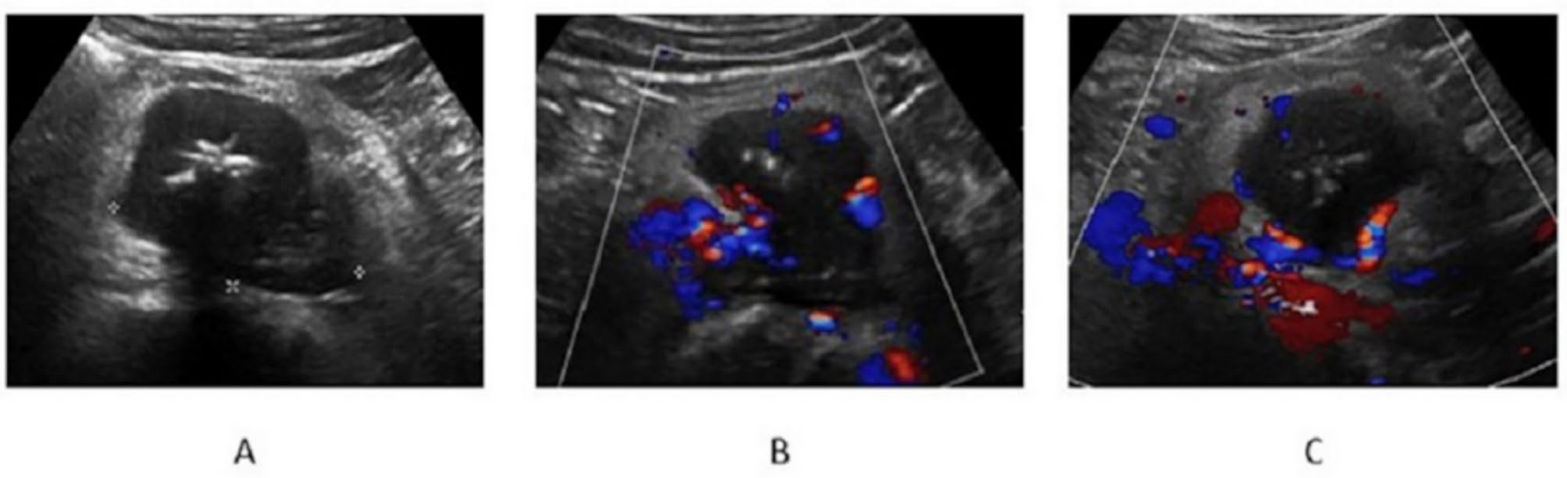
US images from a 36-year-old man with a palpable mass in the right lower quadrant. The pathological diagnosis of the enlarged LN was the mixed type (A- B) The enlarged lymph node grayscale US. The retroperitoneal lymph node presented 6.1 × 5.1 × 4.4 cm in size. A macro strong echo with the posterior shadow is visible in the enlarged LN. (C) The color Doppler image. The LN showed abundant hilar color flow US, ultrasound; LN, lymph node; cm, centimeter
